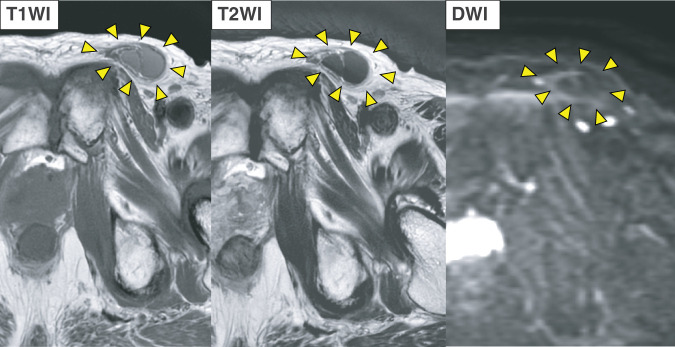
MRI findings. A lobulated 33 mm mass at the entrance of the inguinal canal is visualized (arrowhead). The lesion demonstrates low signal intensity on T2-weighted imaging, intermediate to mildly high signal intensity on T1-weighted imaging, and no restricted diffusion on diffusion-weighted imaging. DWI, diffusion-weighted imaging; T1WI, T1-weighted imaging; T2WI, T1-weighted imaging
